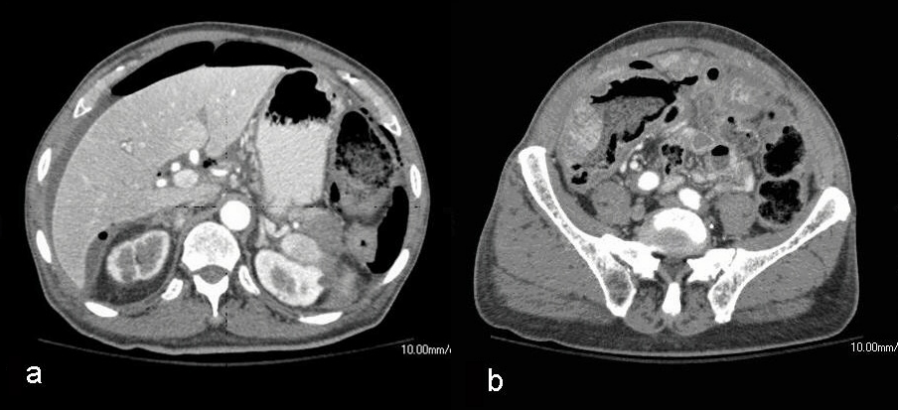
Initial abdominal CT demonstrating free air and fluid and cecal mass; mass in tail of pancreas not initially noted.
